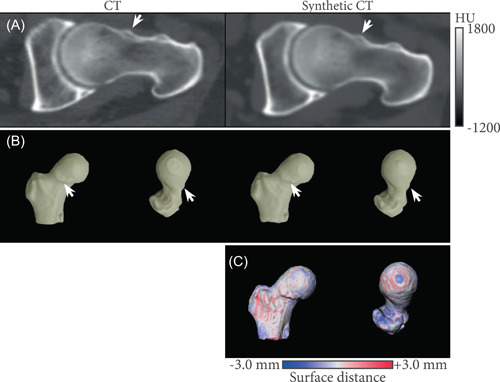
Qualitative comparison between CT and sCT. (A) Radial CT and sCT‐ based 3 o'clock reformats of the left femur obtained for one patient (where 12 o'clock indicates the superior location of the acetabulum and 3 o'clock indicates its anterior location). (B) Corresponding three‐dimensional (3D) femur reconstructions as seen from anterior and superior locations. White arrows indicate a bump around the femoral neck, with good correspondence between CT and sCT images and bone 3D renderings. (C) Surface distance from the sCT bone model to the CT bone model mapped on the sCT bone model. Negative values indicate the sCT model is larger. CT, computed tomography; sCT, synthetic computed tomography [Color figure can be viewed at wileyonlinelibrary.com]
